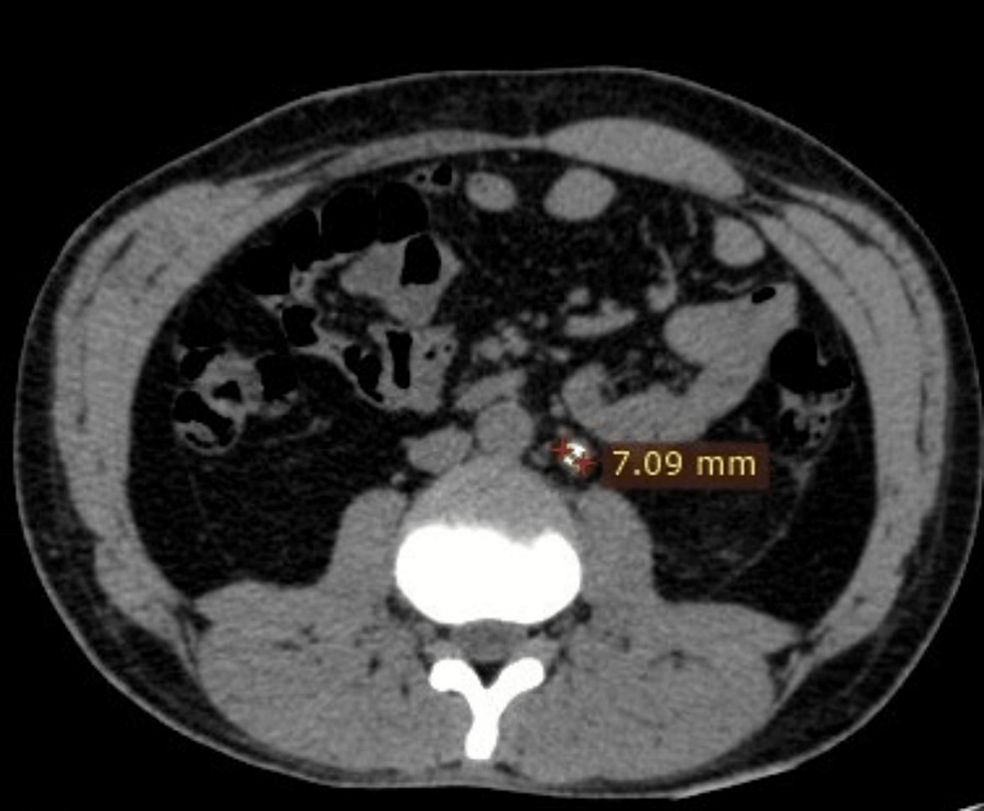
Axial CT section of the abdomen shows a left ureteric calculus and its dimensions.
